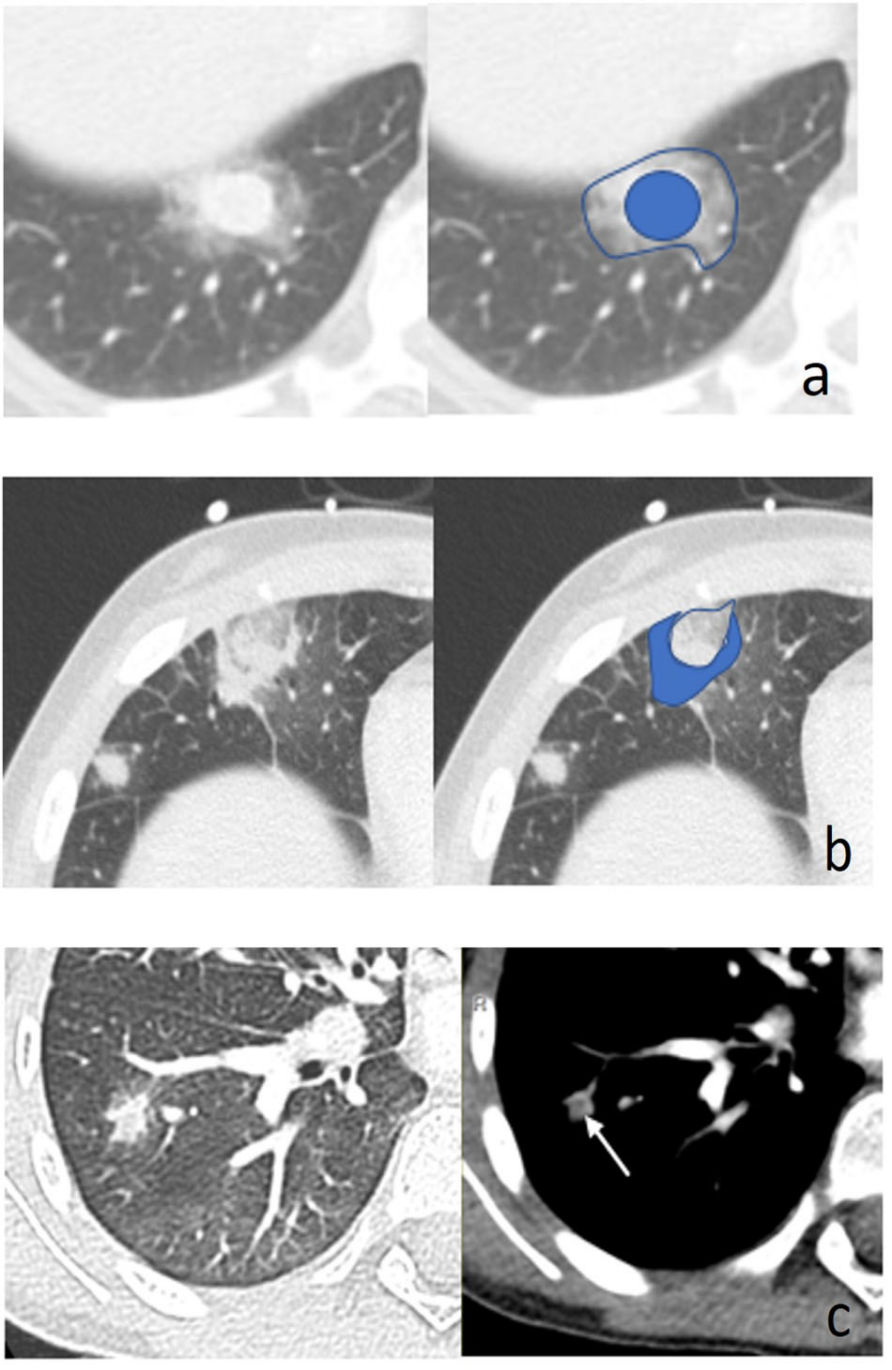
Chest computed tomography (CT) imaging signs suggesting invasive fungal infection (IFI). Axial chest CT images show the “halo sign” (a), consisting of a solid lung nodule (in blue) surrounded by a ring of ground glass in the right lower lobe. The “reversed halo sign” (b) appears as an area of ground-glass opacity surrounded by an almost complete ring of pulmonary consolidation (in blue) in the middle lobe. The “hypodense sign” (c) shows central necrosis (arrow) in a solid nodule in the right lower lobe
